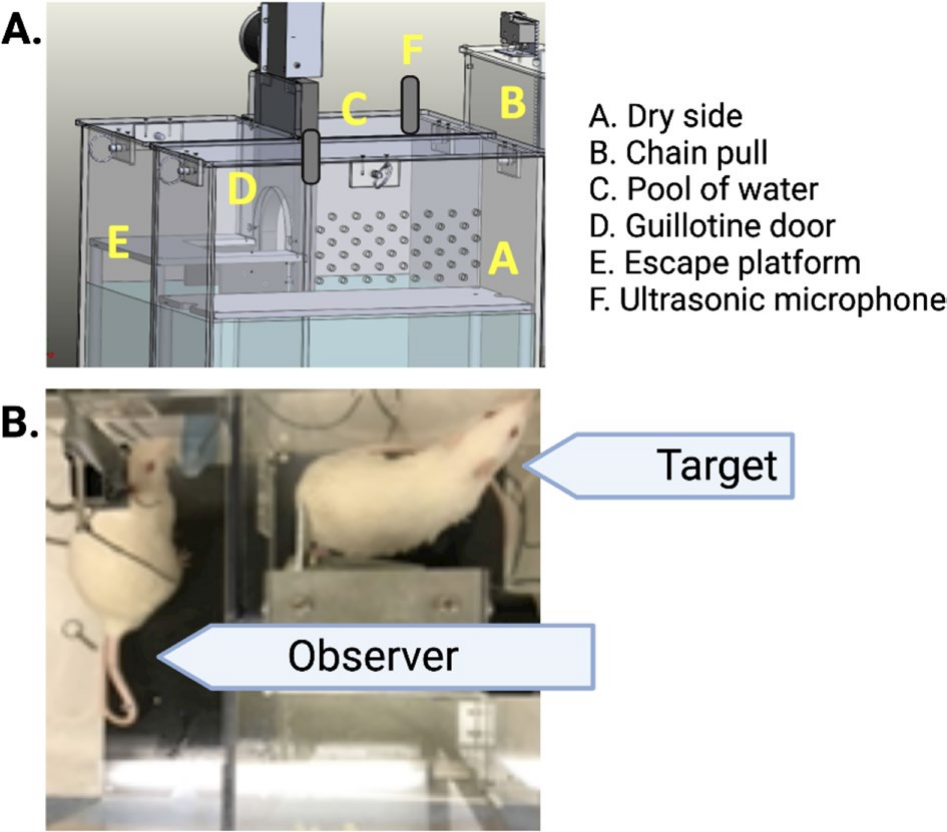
The insula is active during targeted helping. (A) Depiction of the apparatus used during the social contact-independent targeted helping task. Observers pull a chain that opens an automated guillotine door, allowing the Target to escape the distressing context into a separate dry platform. Ultrasonic vocalizations were recorded in some of the following experiments using two high quality condenser microphones attached to the top of the dry and water compartments. (B) This photo depicts an Observer in the dry side of the apparatus and a Target on the escape platform after being released from the pool of water.
